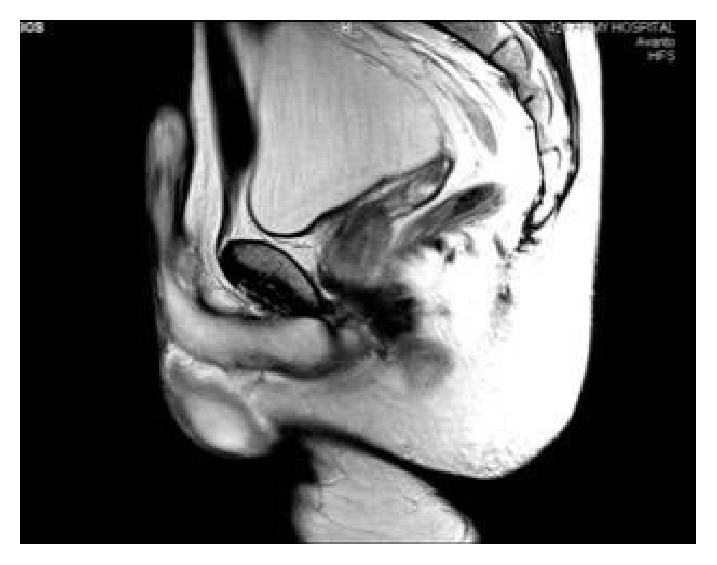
MRI of the penis T2 sagittal, demonstrating a distinct nodule on the dorsal aspect of the penis.
